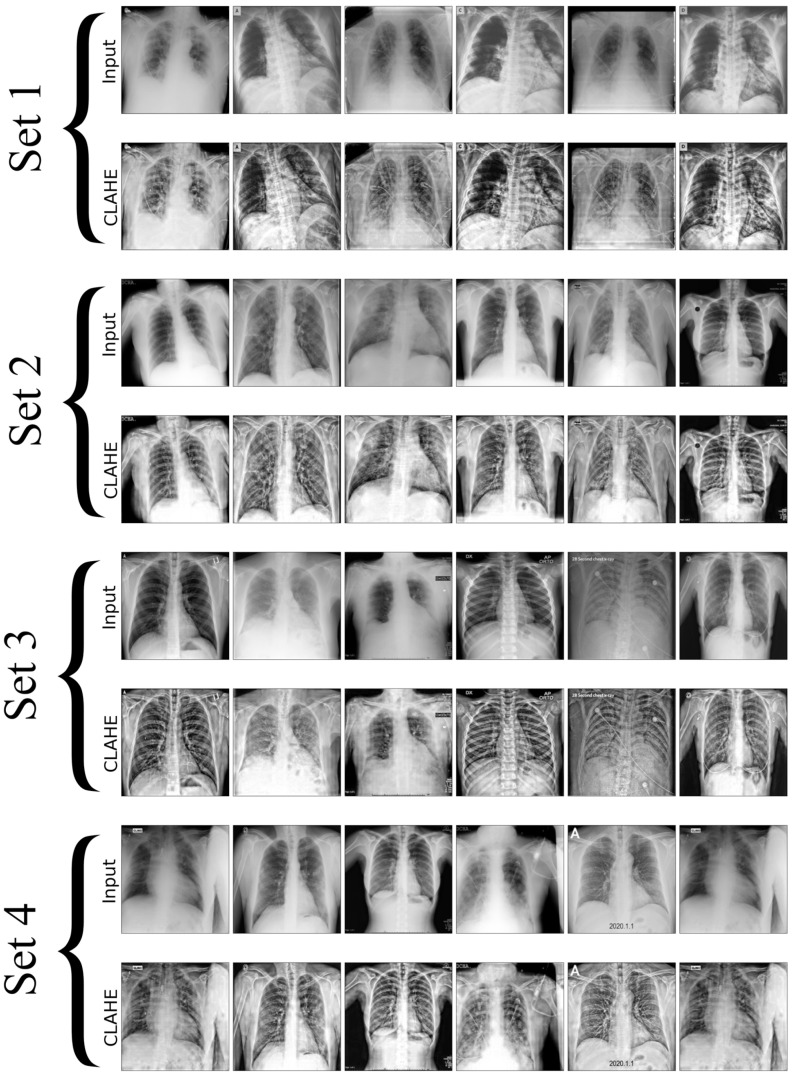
Side-by-side comparison of a chest X-ray before and after CLAHE enhancement.
